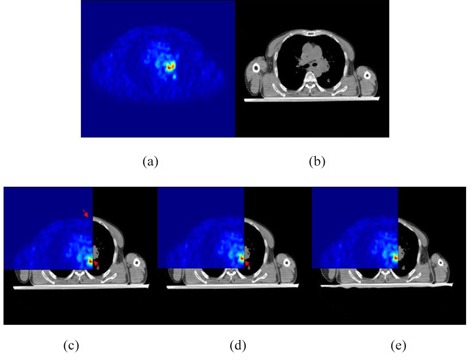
Split window display of PET and CT images: (a) PET image; (b) CT image; (c) overlaid PET‐CT image without any registration; (d) overlaid PET‐CT image with global registration; (e) overlaid PET‐CT image with GMI demons method registration. After precise registration, the two images match each other well. Red arrows indicate the places of larger errors.
